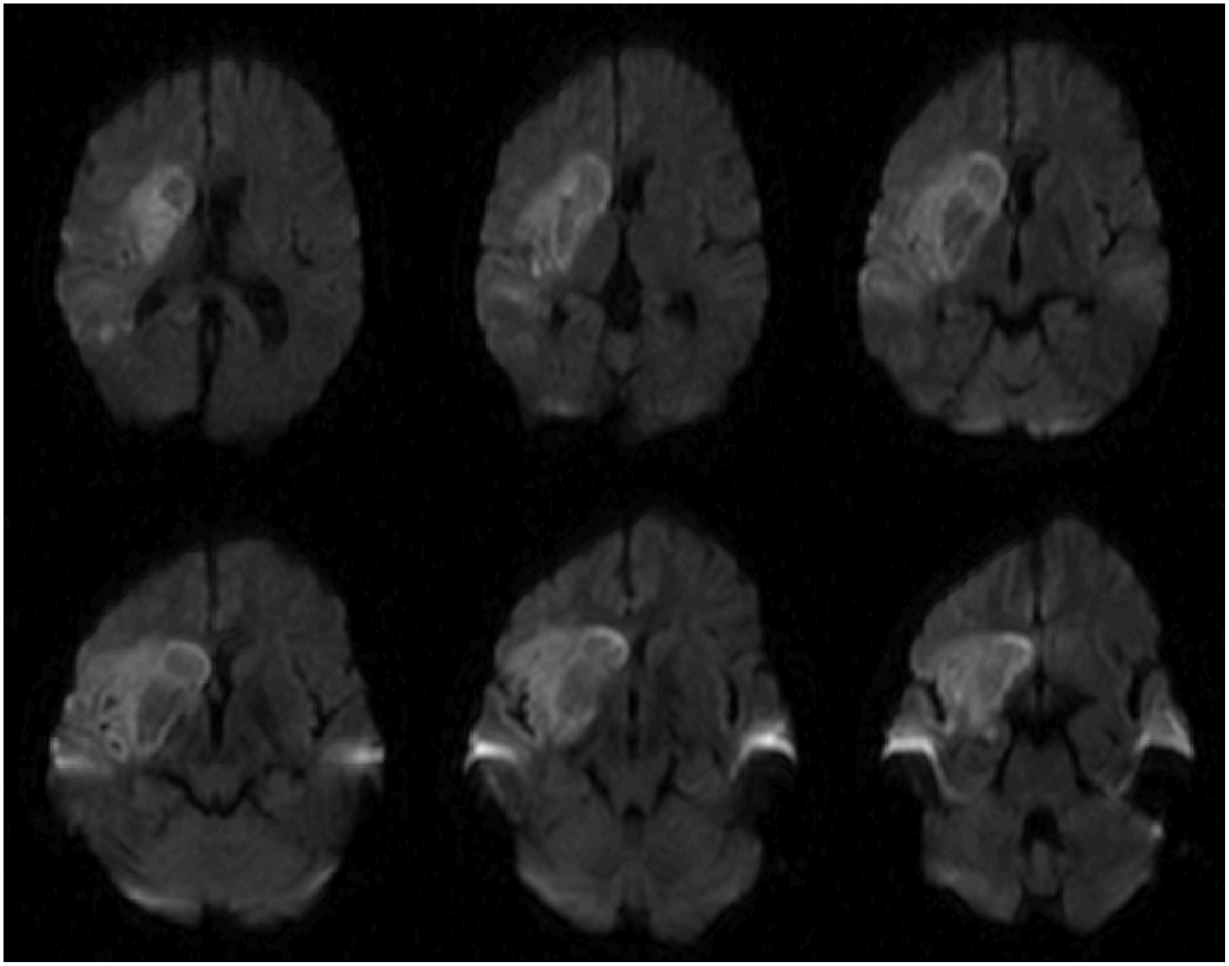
Diffusion weighted imaging (DWI) sequences demonstrating diffusion restriction in the basal ganglia that is characteristically seen in rhinocerebral mucormycosis.
